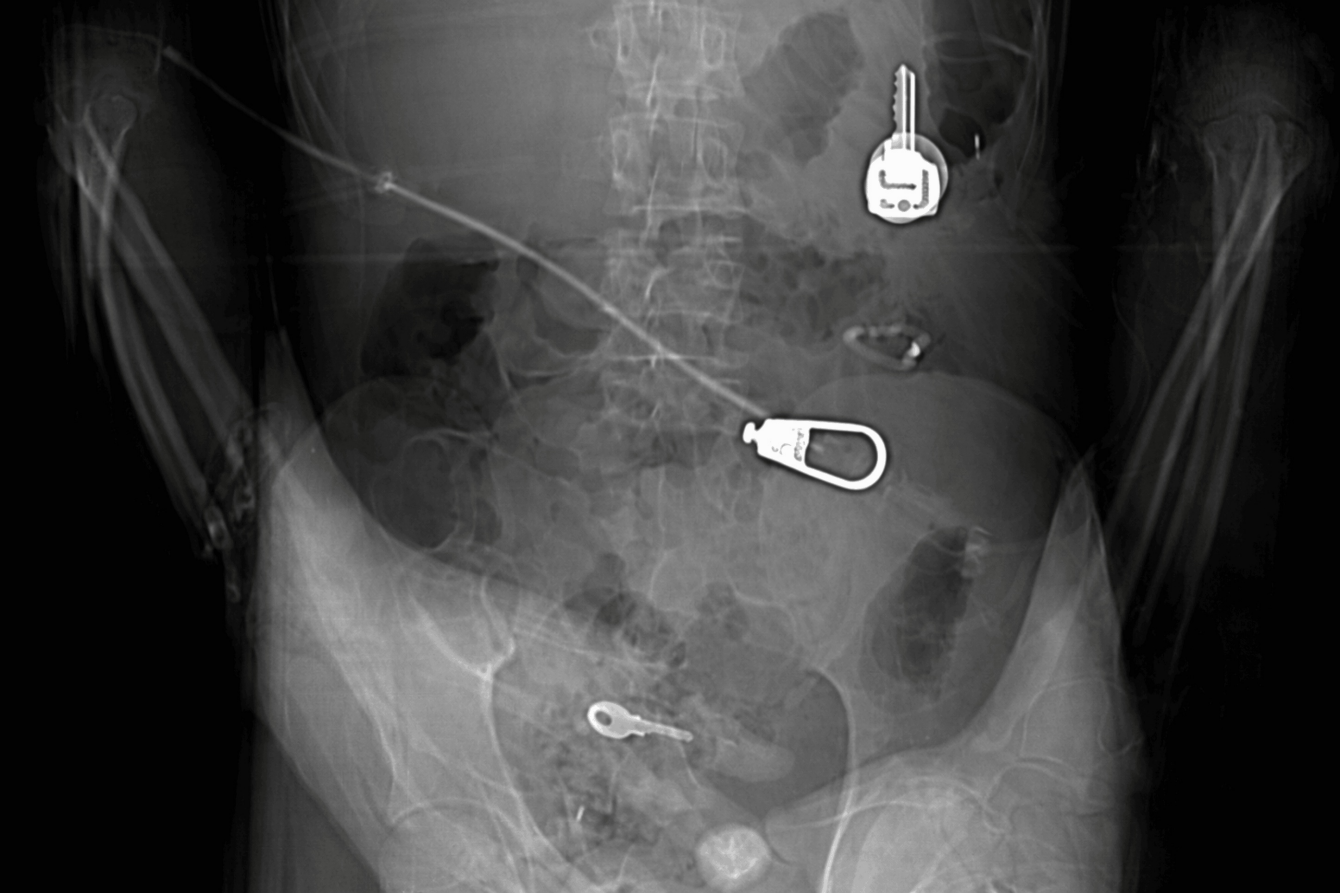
Anteroposterior CT scout image showing multiple radiopaque foreign bodies throughout the gastrointestinal tract.
